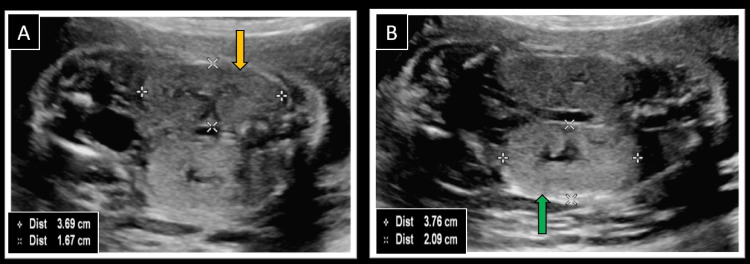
Ultrasound images showing measurements of fetal kidneys Coronal ultrasound images showing length and width measurements of (A) Right fetal kidney (yellow arrow) = 3.69 x 1.67 cm and (B) Left fetal kidney (green arrow) = 3.76 x 2.09 cm, both of which were enlarged for gestational age (Z-score greater than two standard deviations) [7]. Note the normal shape and contour of both kidneys, with no evidence of any mass lesion or cyst.
